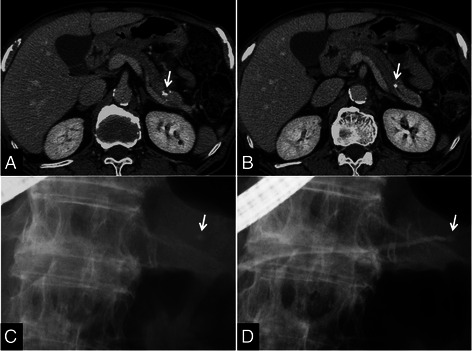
CT and ERCP findings in a 71-year-old man who experienced a pancreatic attack relapse and was treated with ESWL. (A), (B) Contrast-enhanced CT before ESWL showing the pancreatic stone (arrows). (C), (D) ERCP before ESWL identifying the obstructing X-ray-positive stone in the MPD (arrows). Pre-pancreatograpy (C) and post-pancreatography (D) images.
